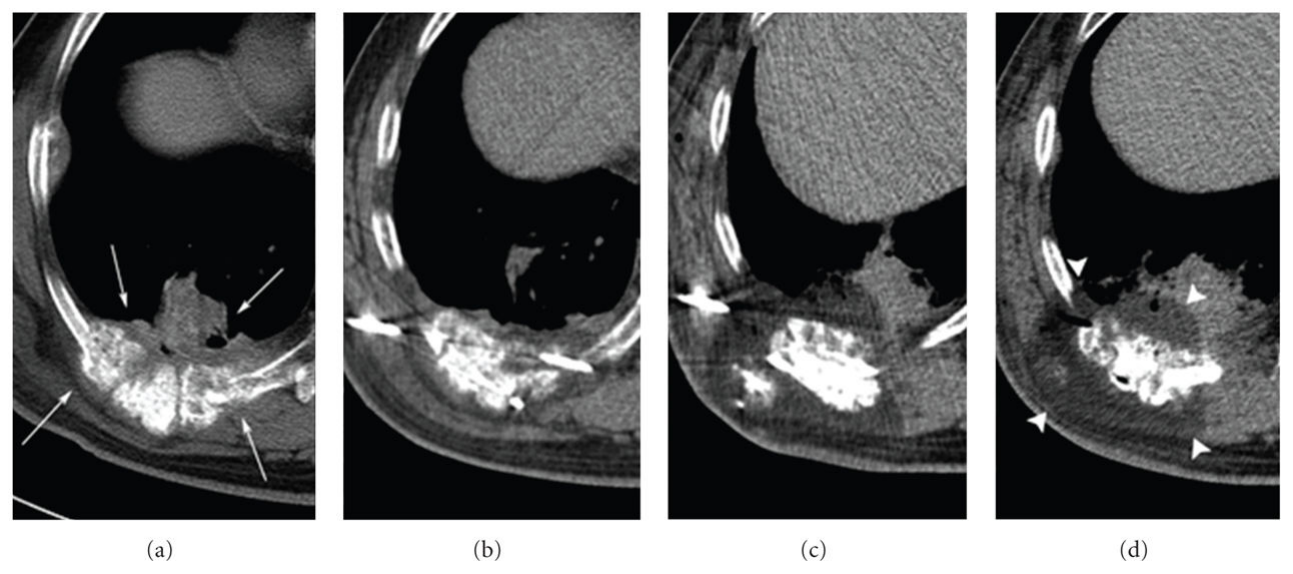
64-year-old male with metastatic colon cancer with prior RF ablation of a hepatic lesion presents with chronic pain from 4 right posterior chest wall masses involving the ribs, pleura, and adjacent musculature containing diffuse calcifications. Axial CT images (from left to right) demonstrate the total area of these 4 abutting masses to measure approximately 10 × 6.5 × 10 cm (a). A total of eight 2.4 mm cryoprobes were utilized during the procedure, with seven probes initially placed for the first freeze cycle, and an additional eighth probe was placed to cover the superficial/lateral tumor margin in the second freeze cycle ((b) and (c)). Up to 60 cc of saline were continuously injected to protect the overlying skin from the ablation zone. Final ice formation appeared to cover all tumor margins and measured 12 × 8 × 12 cm (d).
